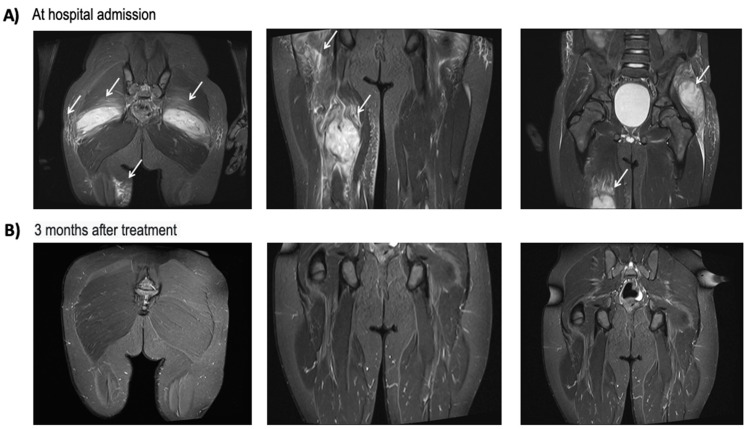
Imaging of subcutaneous and muscular abscesses caused by PVL-positive MRSA infection. Magnetic resonance imaging (MRI) scans of a 9-year-old child (Patient #2) admitted for multiple bilateral subcutaneous and muscular abscess collections (white arrows) localized to the thigh and gluteal region, attributed to PVL-positive *S. aureus* (Panel A). The patients underwent targeted antibiotic treatment for 3 weeks followed by a single infusion of dalbavancin. Three months post-treatment, a new MRI (Panel B) revealed complete resolution of subcutaneous and muscular lesions.
